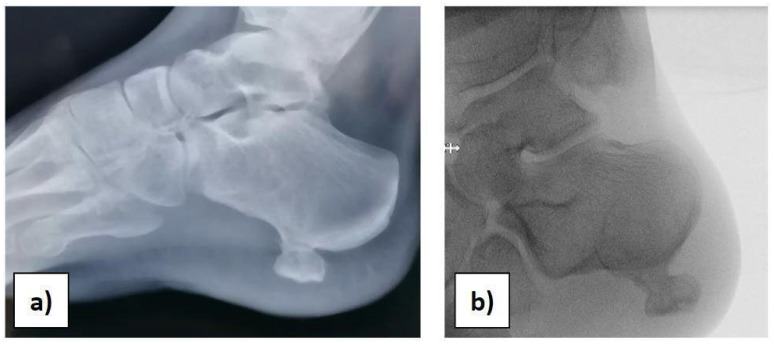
(a) Preoperative X-ray. (b) Intraoperative X-ray before starting the surgery.
